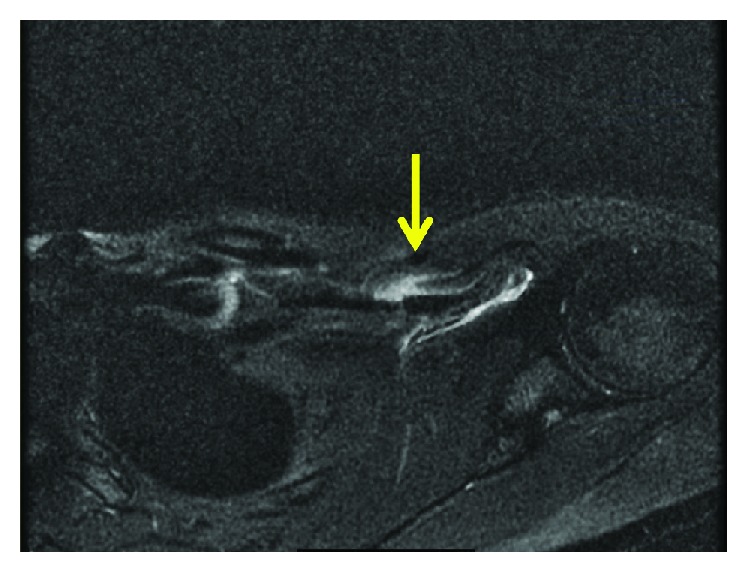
Axial T2-weighted magnetic resonance imaging showing disruption of the pectoralis minor myotendinous junction with surrounding edema and associated intramuscular edema.
